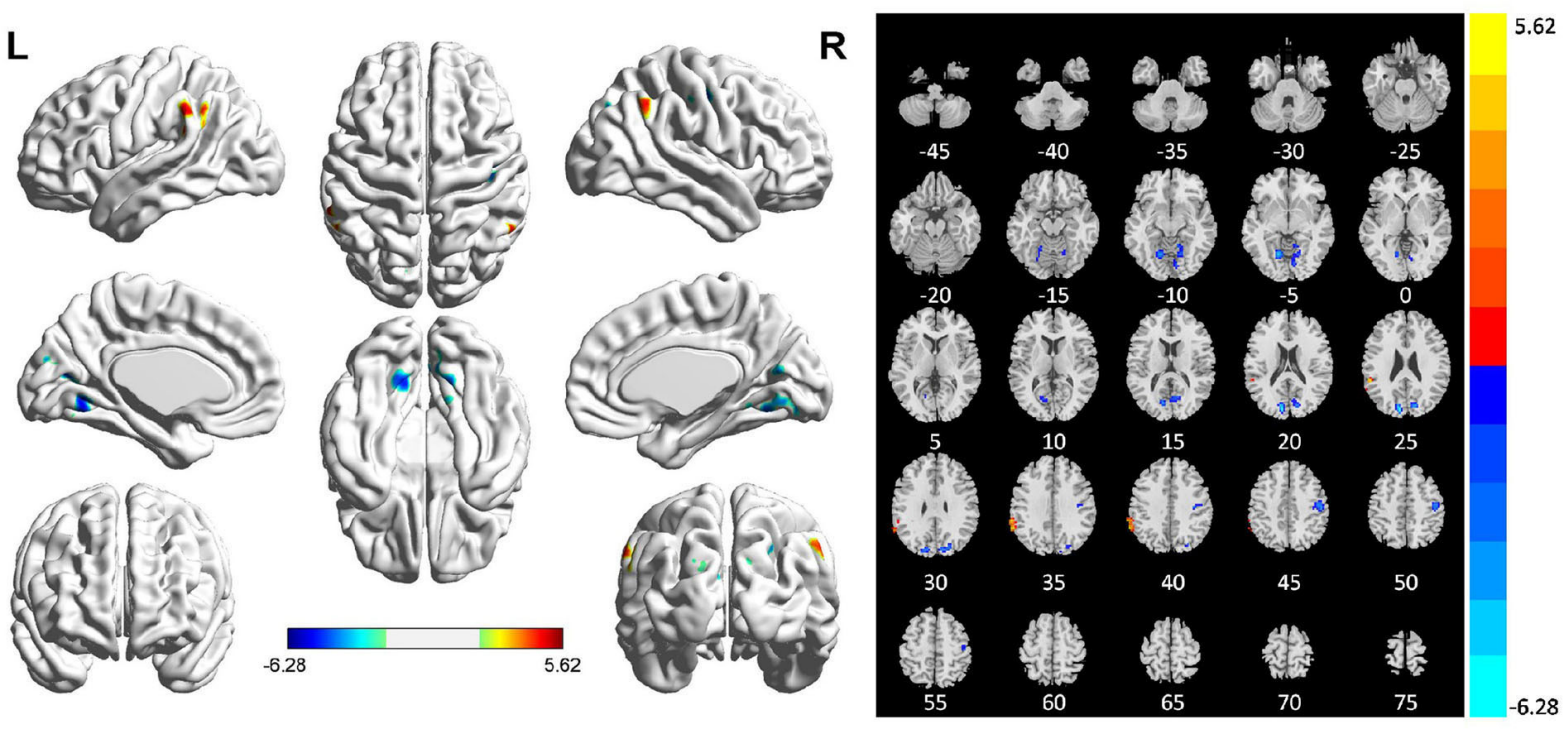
Statistically significant differences between patients with post-stroke insomnia (PSI) and healthy controls are shown in a regional homogeneity (ReHo) map of the whole-brain with magnetic resonance imaging (MRI). Patients with PSI showed a significant ReHo increase in the left supramarginal gyrus (warm colors), as well as a decrease in the bilateral lingual gyrus, right cuneus, right precentral gyrus, and posterior central gyrus with decreased ReHo values (cold colors). A T-score bar is shown below and right. Red and blue denote increases and decreases in ReHo, respectively.
